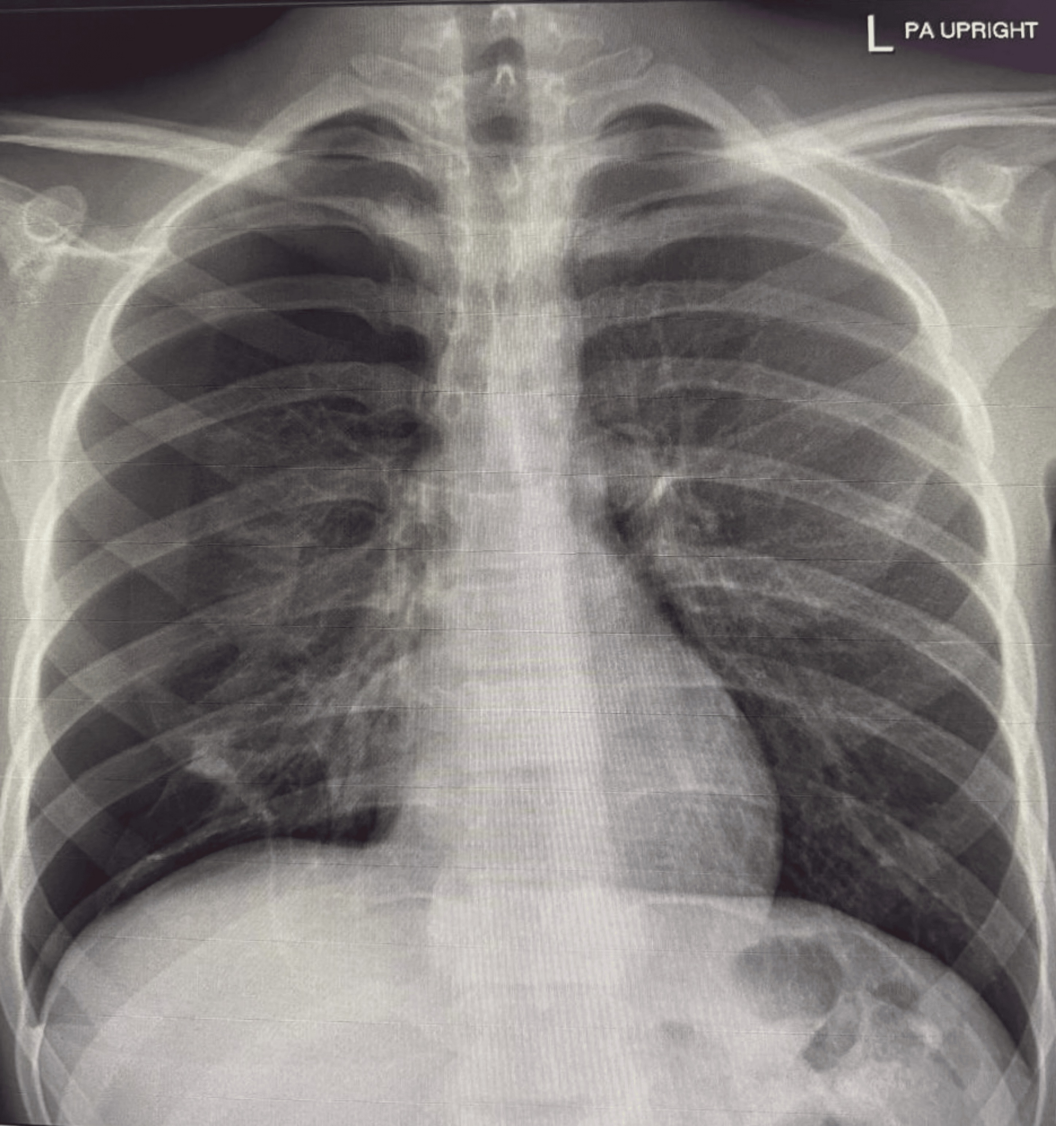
Chest X-ray posteroanterior (PA) view.
